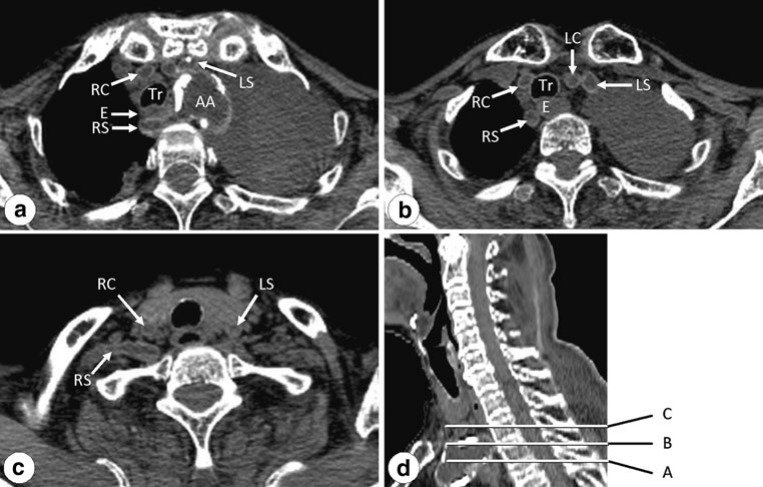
Computed tomograph images of horizontal sections at the level of the third thoracic vertebra (a), at the boundary between the second and third thoracic vertebrae (b), and at the second thoracic vertebra (c). d A sagittal section indicating the horizontal section levels of CT images in a, b, and c (*A*,* B*, and* C*, respectively). The right subclavian artery can be confirmed to be crossing between the vertebral body and esophagus (a). *AA* Aortic arch, *E* esophagus, *LC* left common carotid artery, *LS* left subclavian artery, *RC* right common carotid artery, *RS* right subclavian artery, *Tr* trachea
